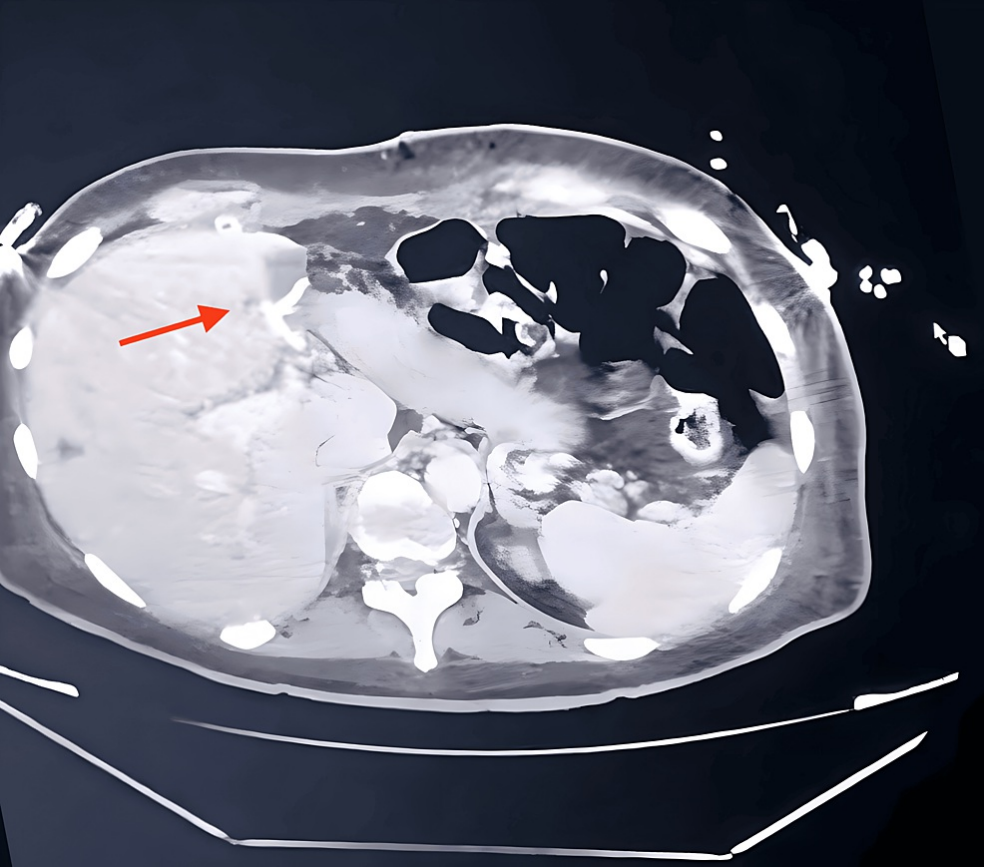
Post-surgical Computed Tomography The red arrow shows segment IVb
